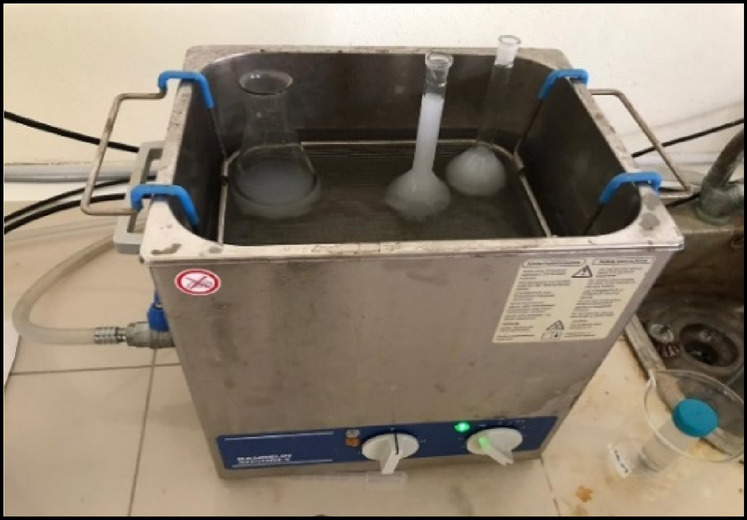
Ultrasonic dispersion of NS in deionized water.
